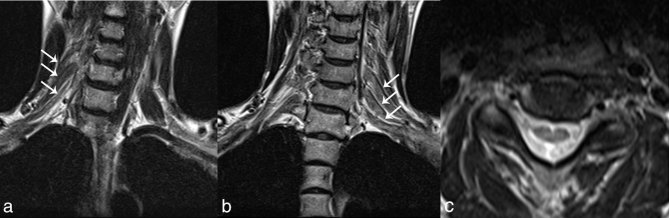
Coronal fast spin-echo *T*_2_ weighted MR images show enlargement of the bilateral brachial plexus (arrows in a and b). Axial fast spin-echo *T*_2_ weighted MR image demonstrates atrophy of the cervical spinal cord (c).
